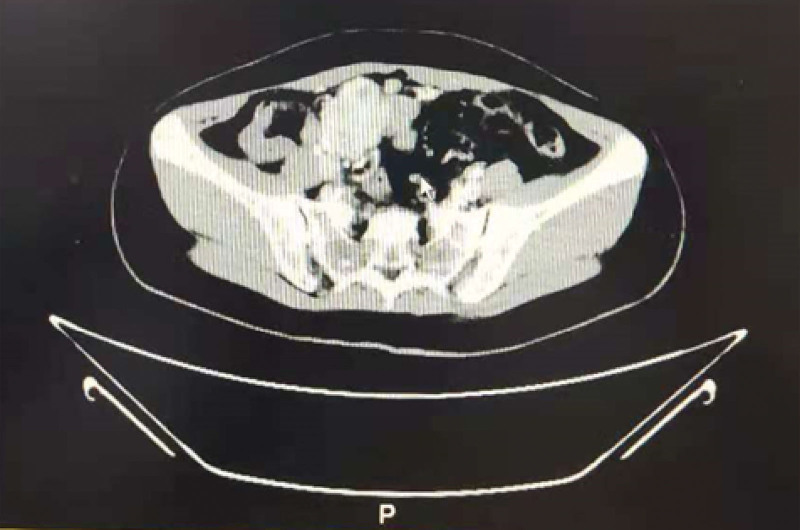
Enhanced computed tomography scan revealed irregular blood-supplying masses in the abdominal and pelvic cavity.
